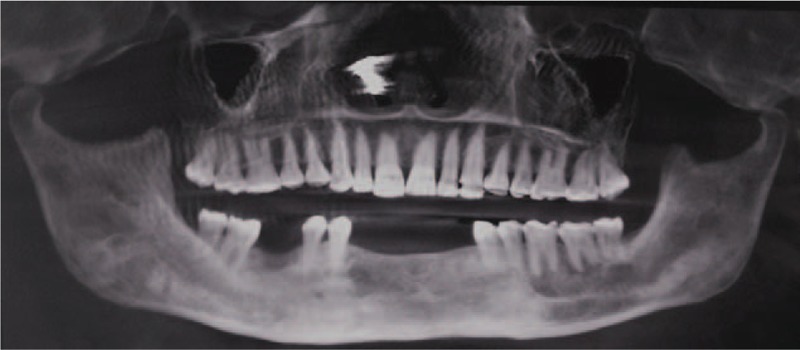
Postoperative radiograph at a 10-year follow-up showing complete resolution of the lesion as evidenced by replacement of radiolucency by radiopaque bone.
